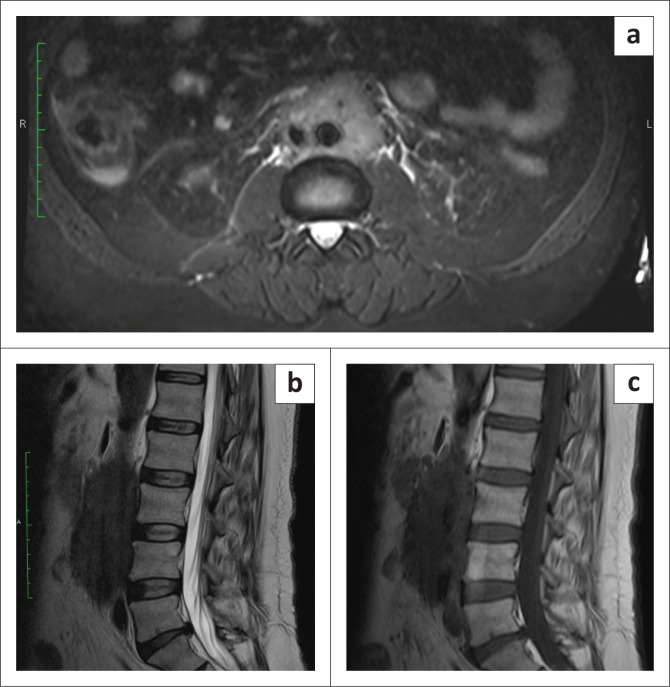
Axial (a) and sagittal (b–c) MR images of the lumbar spine of case 2 show a short tau inversion recovery (STIR) hyperintense (a) and T1-weighted and T2-weighted hypointense (b–c) circumferential mass encasing the abdominal aorta and IVC.
